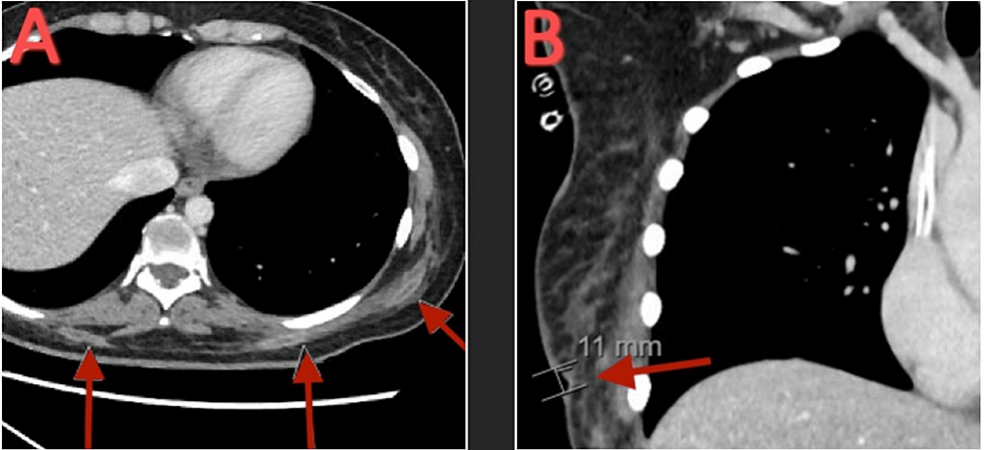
CT thorax with contrast. (A) Transverse view showing multiple tubular, interconnecting, loculated soft tissue abscesses. (B) Coronal view showing a 1.1 cm soft tissue ulcer in the inferior and lateral aspect of the right breast.
